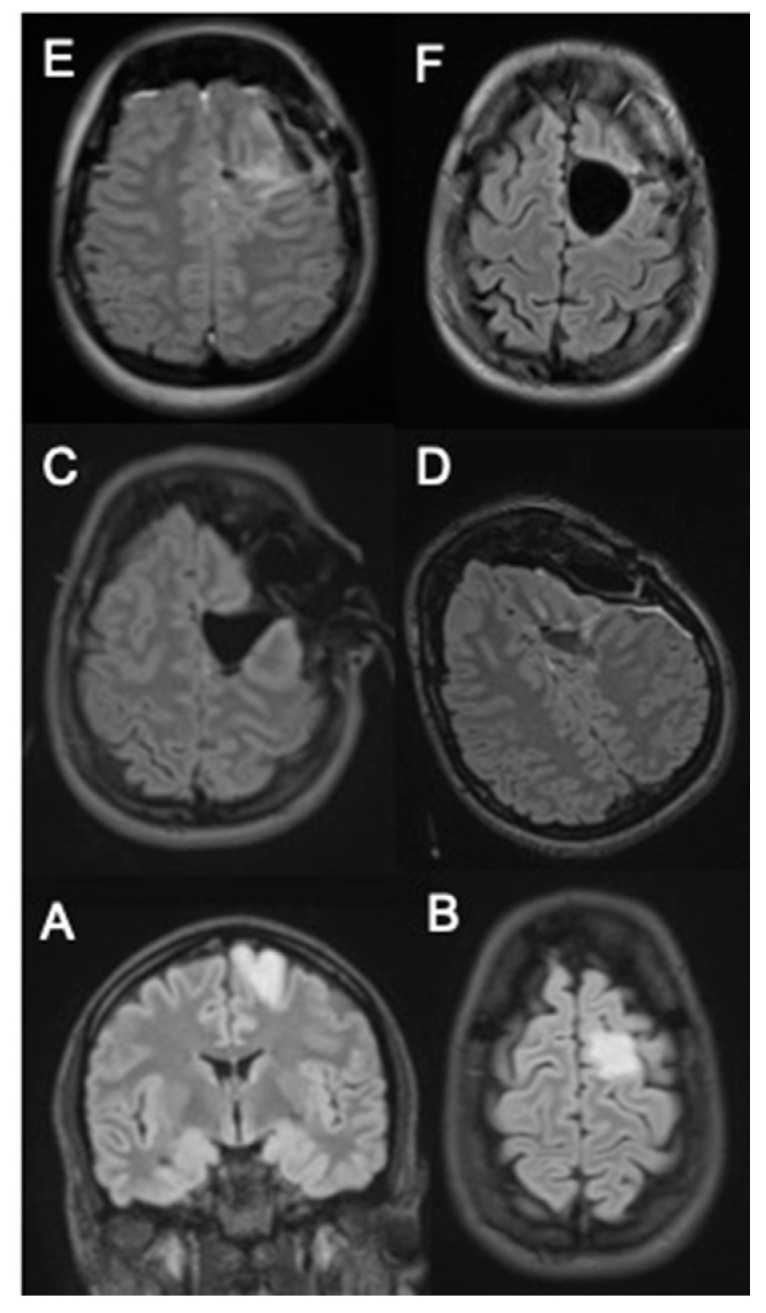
(A,B) hyperintense tumor mass without contrast enhancement on coronar and axial FLAIR. (C) intraoperative and (D) ultra-early postoperative axial MRI FLAIR images. (E) early postoperative axial FLAIR MRI and (F) late axial follow-up FLAIR MRI 3 months after surgery.
